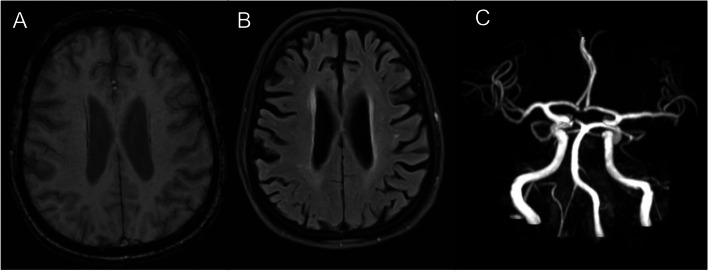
Day 1: post-Thrombectomy Recanalization of the Left Middle Cerebral Artery without Evidence of Hemorrhage or the HARM Sign. A SWAN MRI sequence shows no evidence of post-thrombectomy hemorrhage. B Post-thrombectomy FLAIR MRI sequence shows no evidence of the HARM sign. C 3D time of flight MRI sequence shows post-thrombectomy TICI 3 recanalization of the left middle cerebral artery. FLAIR indicates fluid attenuated inversion recovery; HARM, hyperintense acute reperfusion marker; MRI, magnetic resonance imaging; SWAN, susceptibility-weighted angiography; TICI, Thrombolysis In Cerebral Infarction
